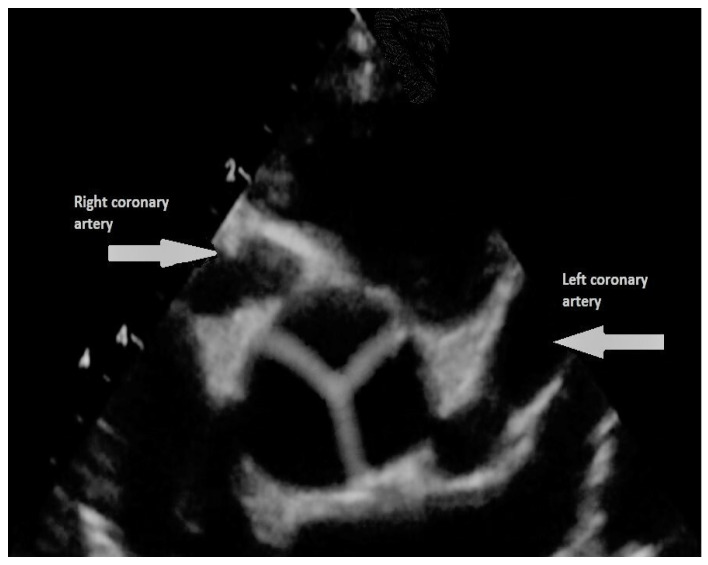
Persisting giant right and left coronary aneurysm (arrows) in a boy with previous Kawasaki syndrome (echocardiographic short-axis view).
